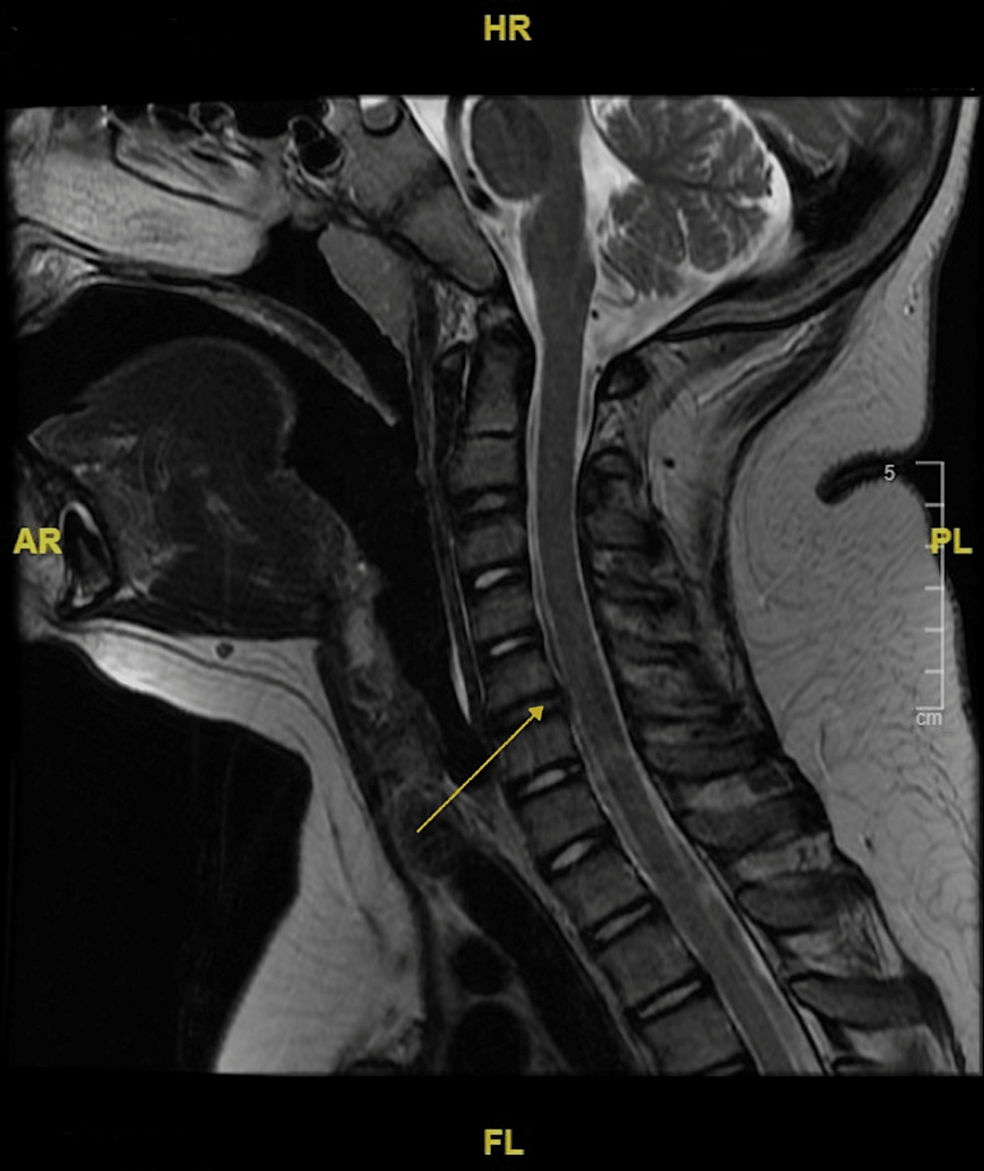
MR cervical spine with contrast shows mild C5-C6 disk bulge The image shows a T2-weighted FLAIR sequence taken on a 1.5-Tesla scanner. Dotarem intravenous contrast (12 mL) was administered using MR cervical spine protocols. There is a normal anatomic alignment of the cervical spine and no evidence of focal disk herniation or cord compression. The intervertebral disc heights and signals are maintained. FLAIR: Fluid-attenuated inversion recovery.
